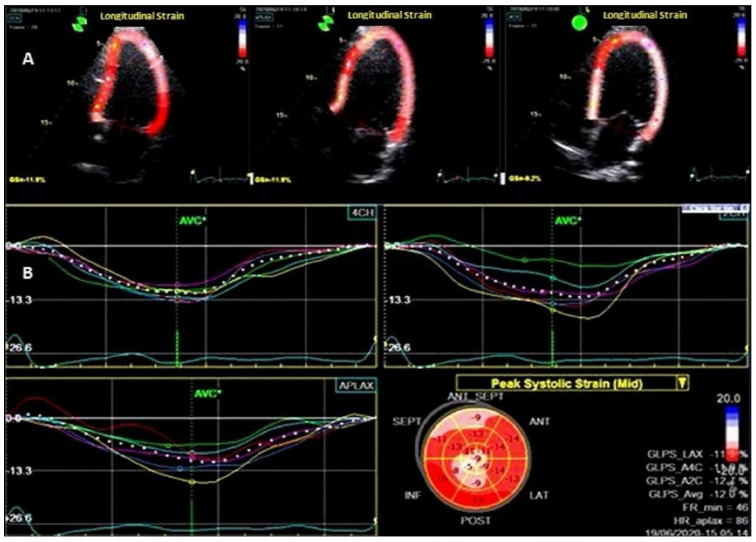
Left ventricular two-dimensional speckle tracking echocardiography. (A) Apical 2, 3, and 4 chamber incidences longitudinal strain analysis; (B) Bull’s eye synopsis.
